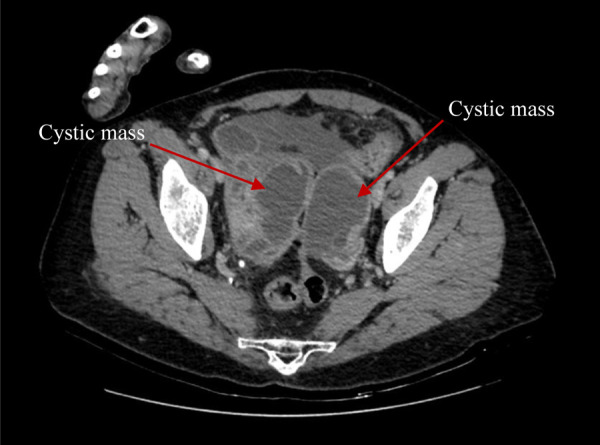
CT report of abdomen and pelvis. Cystic masses in both ovaries (red arrows).
